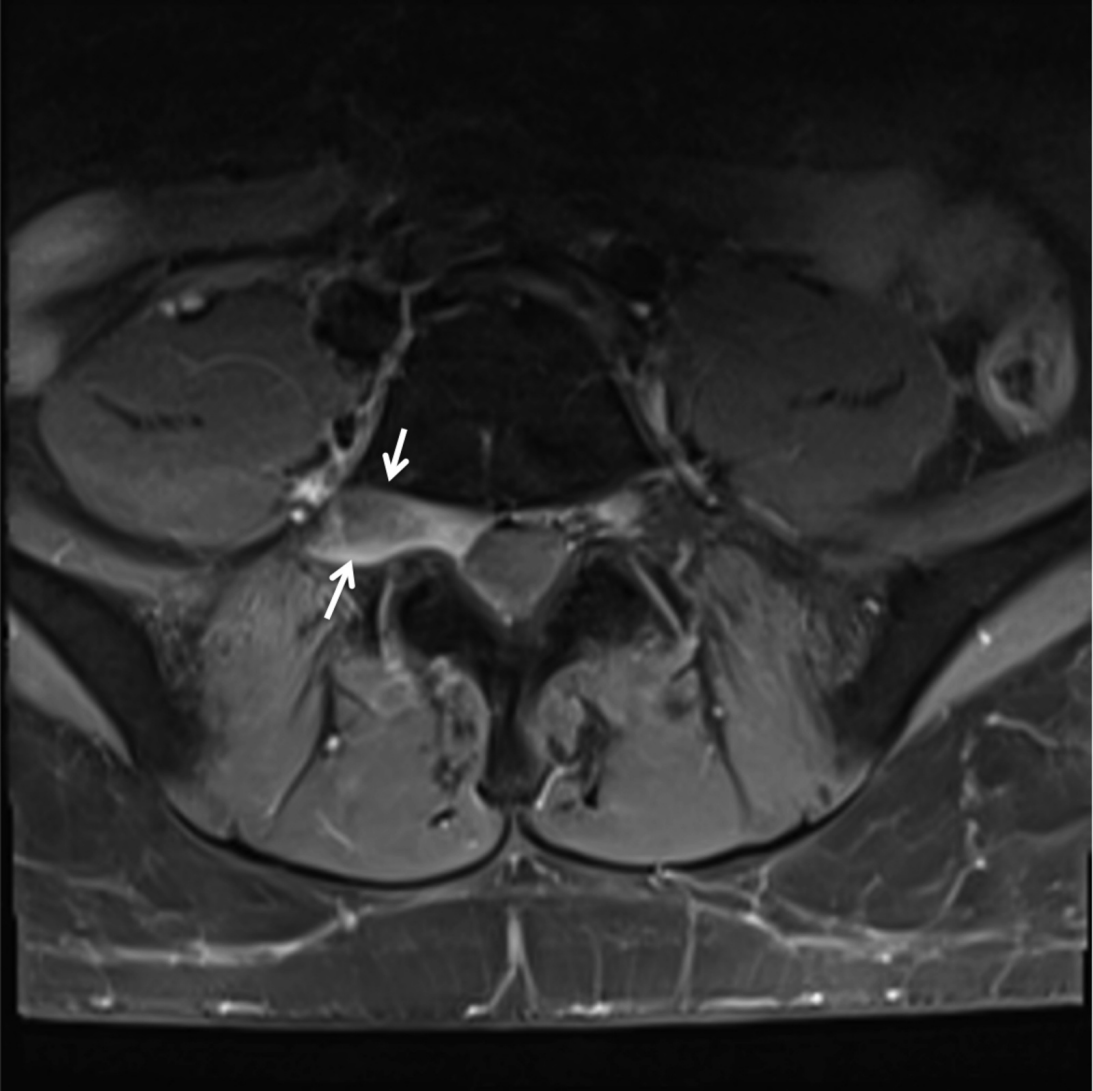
Axial magnetic resonance imaging (MRI) of patient 1. Representative axial MRI of patient 1 noting expansile lesion of right lumbar nerve root.
